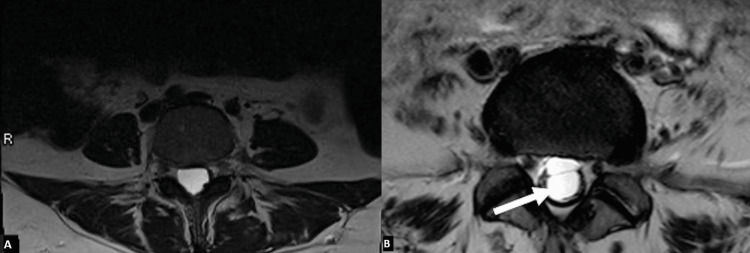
Empty thecal sac sign. Image A is an axial MRI of the spine without contrast, revealing adherent cauda equina nerve roots to the thecal sac, creating an "empty thecal sac" sign in a 33-year-old female patient [12]. Image B is an axial MRI of the lumbrosacral spine examination revealing the distorted nerve roots, adherent to the thecal sac, creating the “empty thecal sac” sign (arrow) typical for adhesive arachnoiditis in a 56-year-old female patient [13].
